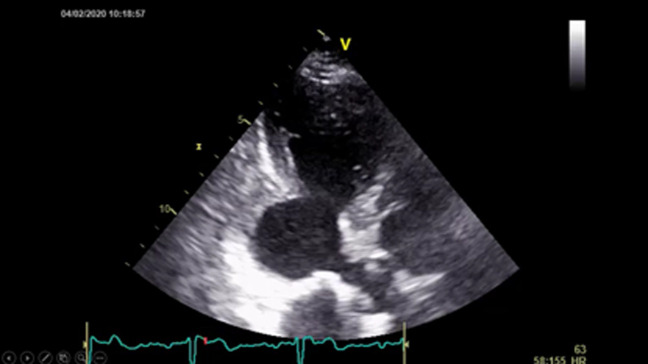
echocardiography in the apical 3 cavities view showing a giant calcified aneurysm extending to the basal inferoseptal wall
